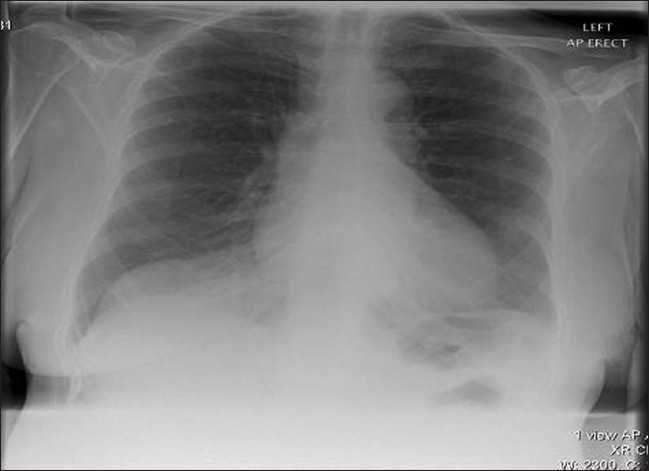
Chest X-ray: Normal with no signs of metastatic lesions
